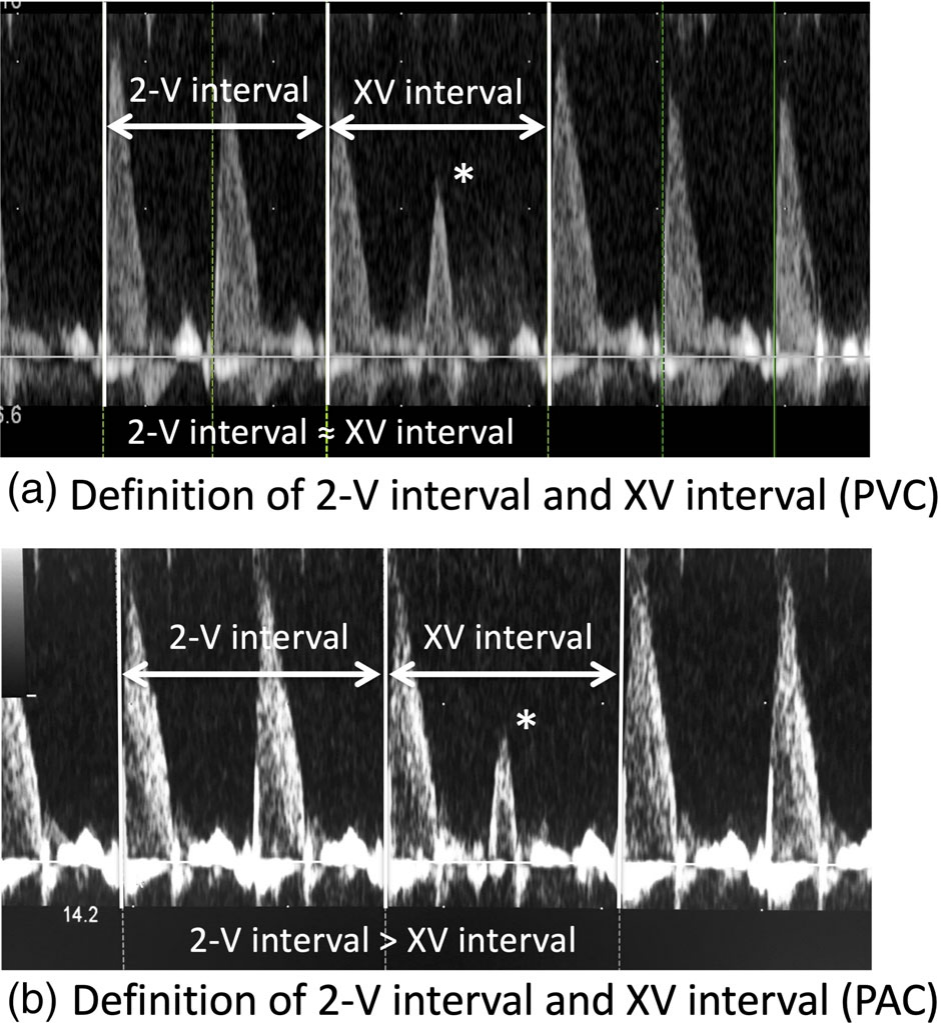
Definition of 2‐V interval and XV interval. The 2‐V interval is the time interval measurement of two consecutive regular arterial Doppler waves just before the premature contraction. The XV interval is the time interval measurement of two arterial Doppler waves, including the premature contraction. (a) A premature ventricular contraction (PVC), and (b) a premature atrial contraction (PAC). For the PVCs, the 2‐V interval was approximately equal to the XV interval. On the other hand, for the PACs, the 2V‐interval was more than the XV interval.
